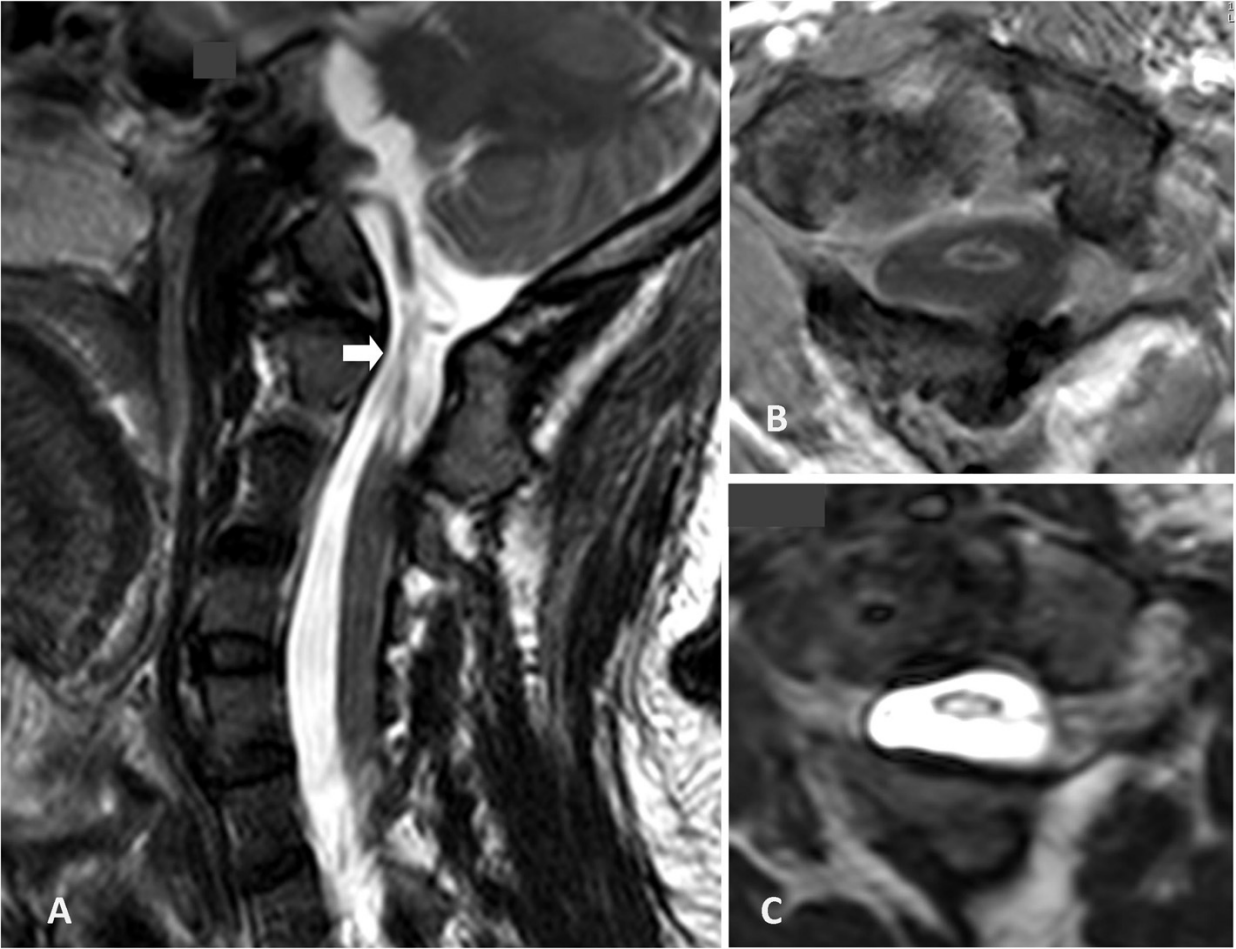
Cervical spinal cord magnetic resonance imaging scan prior to stem cell transplantation. Sagittal and axial T2-weighting (A, C) and axial T1-weighting (B) scans revealed the small diameter of the cervical spinal cord at the C1-C2 level, which demonstrated the atrophic change of the spinal cord (arrowhead).
